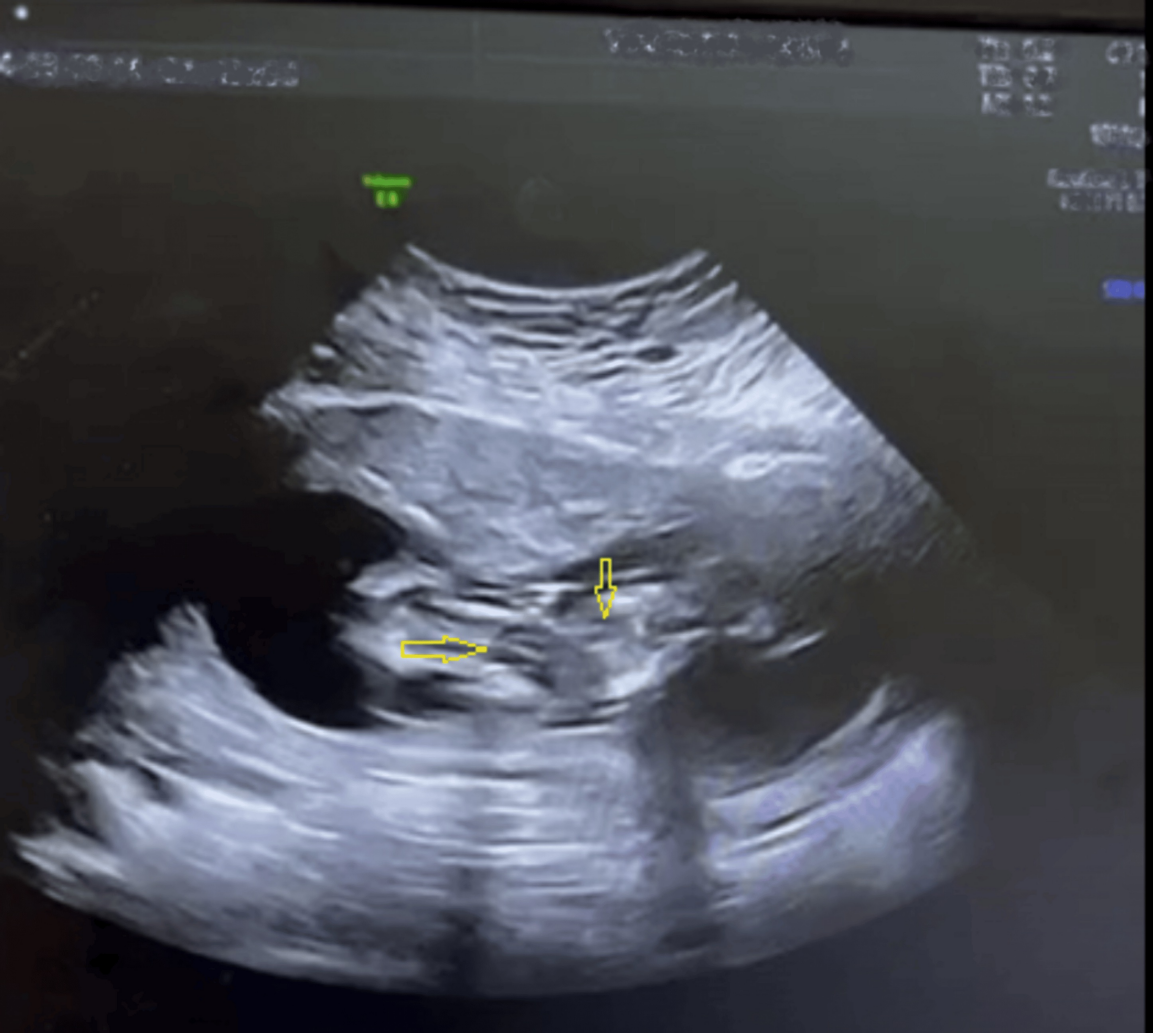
Ultrasound in 13+5 weeks (the yellow arrows show the two distinct hearts)
